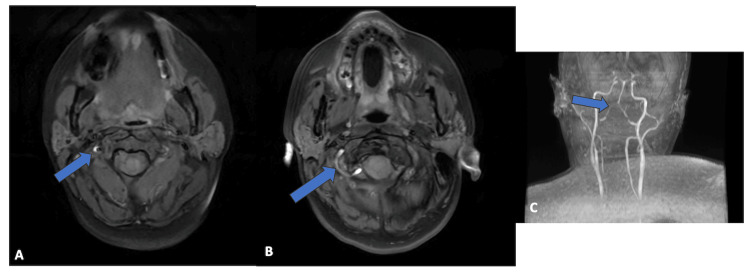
High-resolution T1-weighted fat-suppressed (T1 FS) images (A and B) and 3D time-of-flight magnetic resonance angiography (3D TOF-MRA) image (C) showing eccentric wall hyperintensity and focal thickening of the V3-V4 segments of the right vertebral artery, with luminal narrowing compatible with dissection.
